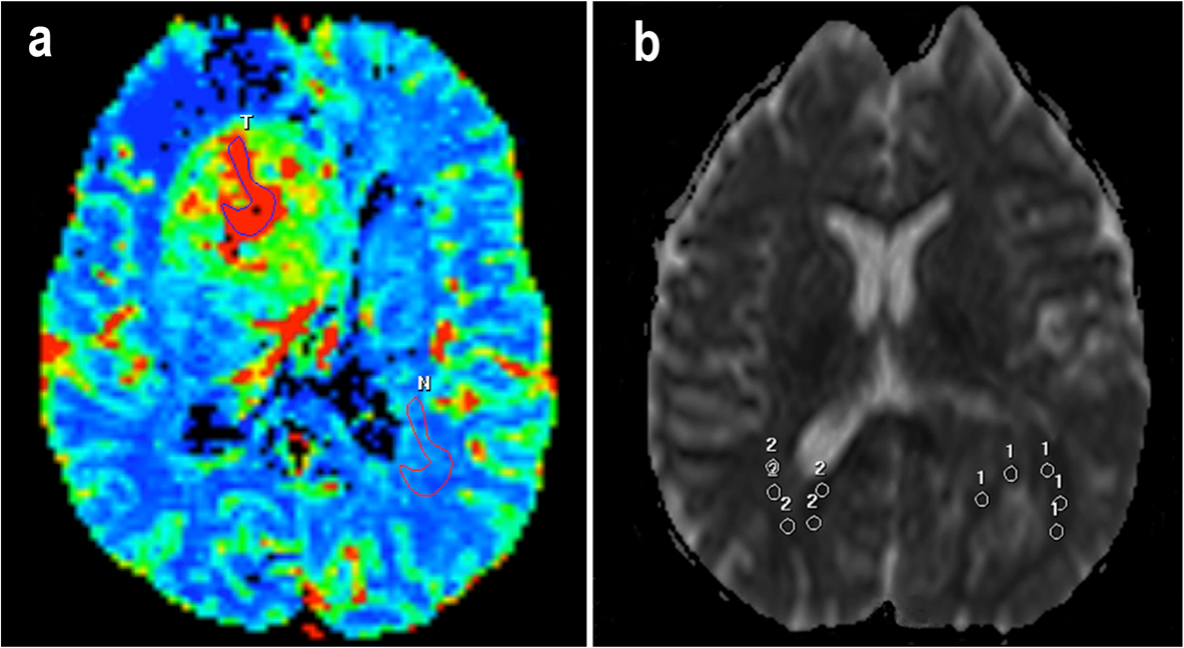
Example of PWI and DWI assessment in cases of glioblastomas (grade IV). a, Calculation of the rCBV ratio. The figure shows the ROI location covering the maximal values of CBV (T) in the parametric map. A similar ROI was placed in the contralateral white matter to normalize the image (N). This image corresponds to the conventional MRI images of Figure 1. b, Calculation of rADC. Example of five different ROIs placed within the solid area of the tumor in the ADC map (represented by number 1) and in the contralateral healthy area (represented by number 2). The MRI features of this case are shown in Figure 2.
